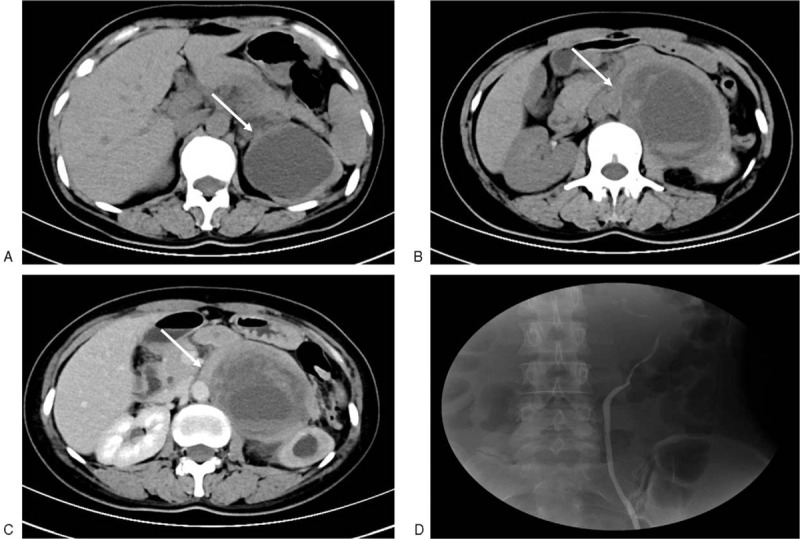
Radiologic features of the retroperitoneal mass. (A) CT scan revealed severe hydronephrosis of the left kidney (arrow head); (B and C) the presence of a 6 × 9 × 8 cm enhancing capsulated solid mass with cystic and necrotic areas in the retroperitoneum(arrow head); (D) left pelvis and upper ureter were not shown in retrograde pyelography.
